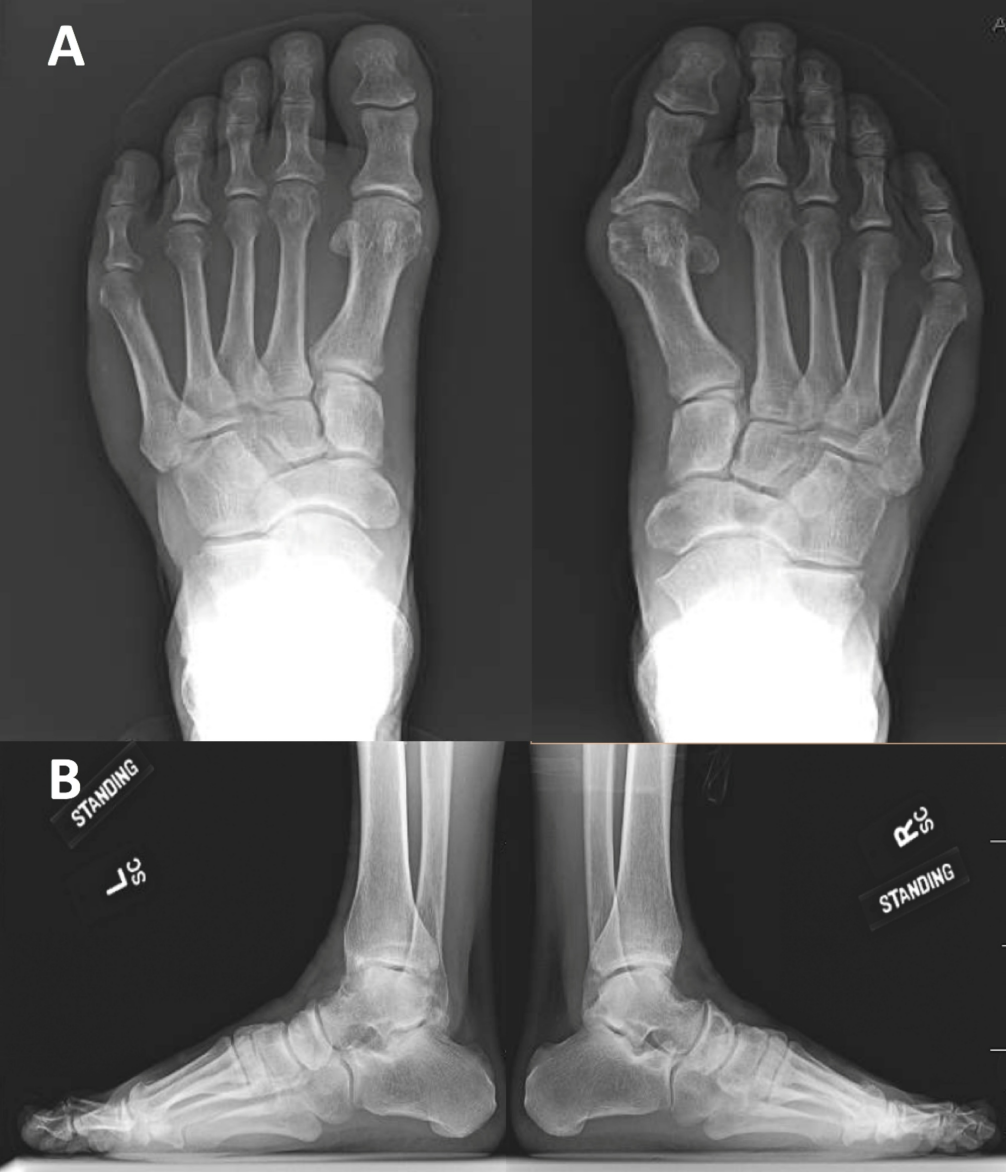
Pre-operative X-ray of bilateral feet, standing: (A) anterior-posterior and (B) lateral views
